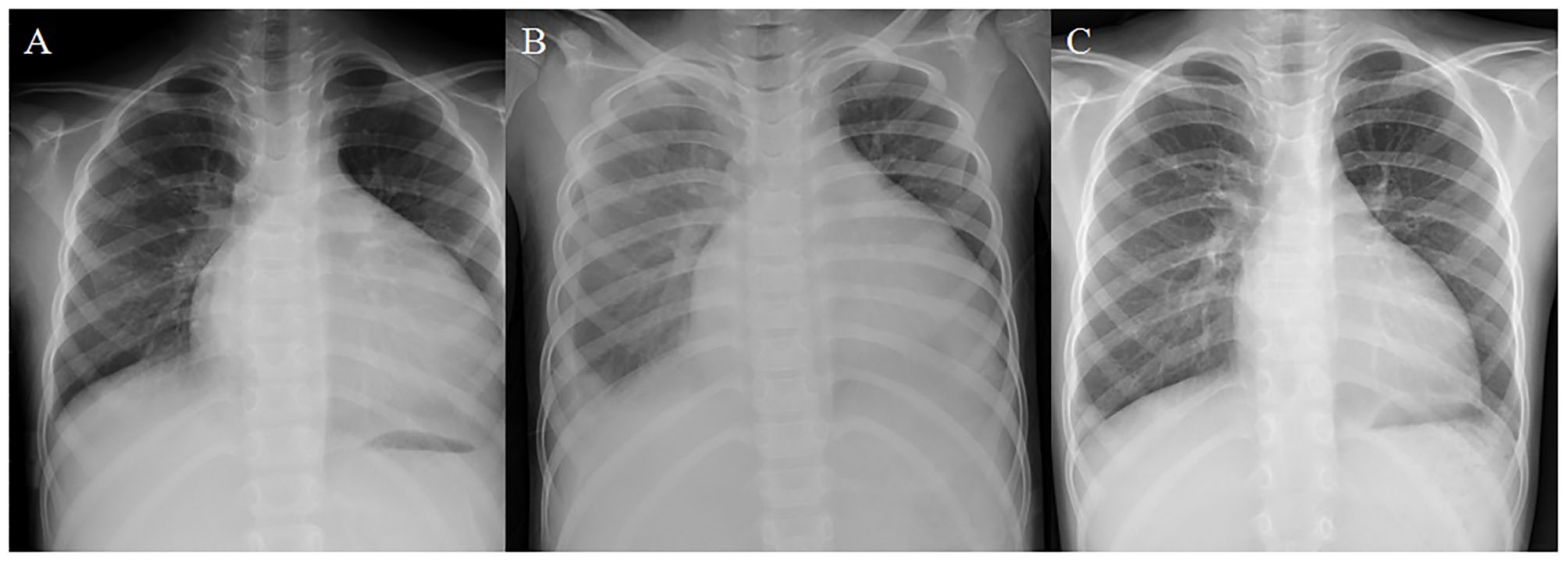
Remarkable improvement in chest X-ray was noted after the initiation of valsartan/sacubitril. (A) Chest X-ray upon admission on the second episode of acute decompensation. The cardiothoracic ratio (CTR) was 0.67. (B) Chest X-ray 2 weeks after aggressive treatment with conventional anti-congestive medications and fluid restriction. Cardiomegaly persisted with progressive right pleural effusion. (C) Chest X-ray 5 weeks after valsartan/sacubitril treatment. The CTR reduced to 0.53, and the pleural effusion had been resolved.
